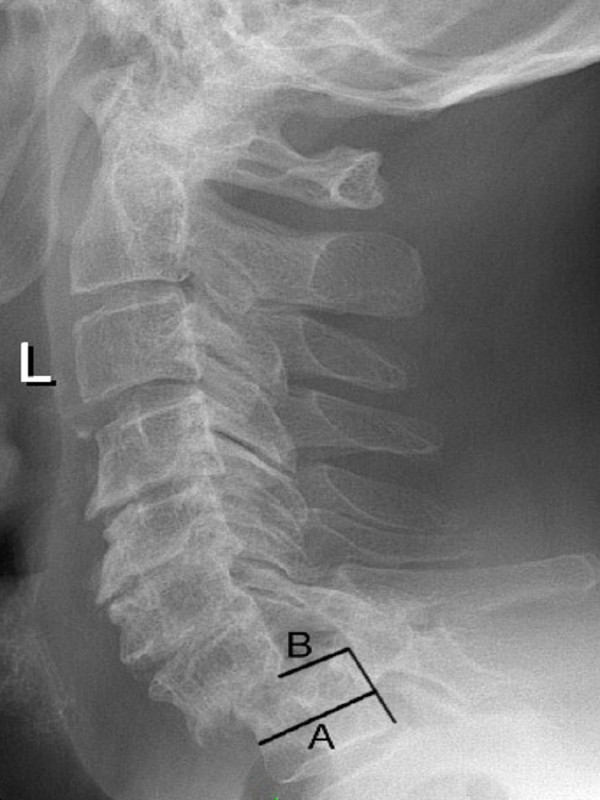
Lateral radiograph of the cervical spine shows a spondylolisthesis at C7-T1 (preoperative); the sagittal diameter of T1 (A) was 22.6 mm and the horizontal displacement (B) between C7 and T1 was 13 mm.
